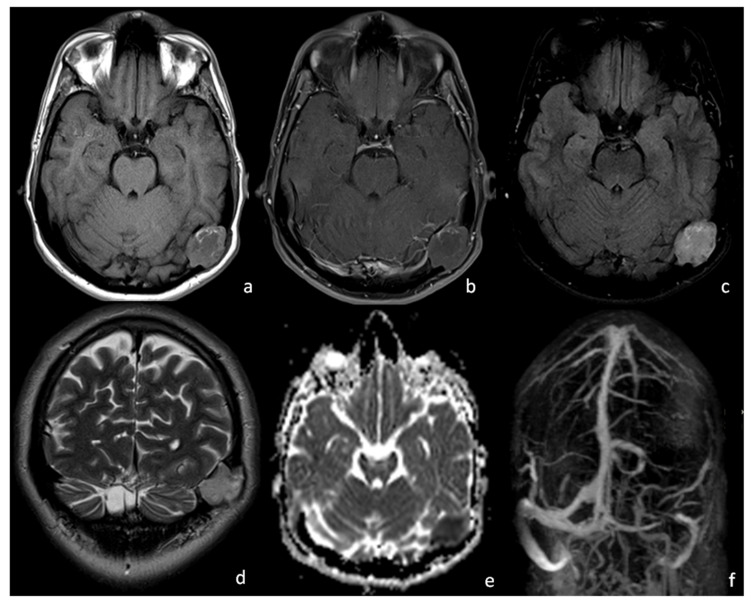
Case 1, 1.5 T MRI: on T1WI (a) there is evidence of a predominantly isointense left asterional mass with a surrounding, subtle, hyperintense rim; no signal enhancement is noted after gadolinium injection (b). The lesion appears to be unevenly hyperintense on FLAIR (c) and T2WI (d), with restricted signal on ADC (e). The 3D MIP reconstruction of contrast-enhanced MR venography (f) shows a severe stenosis of the left transverse–sigmoid sinus junction caused by the asterional dermoid cyst.
